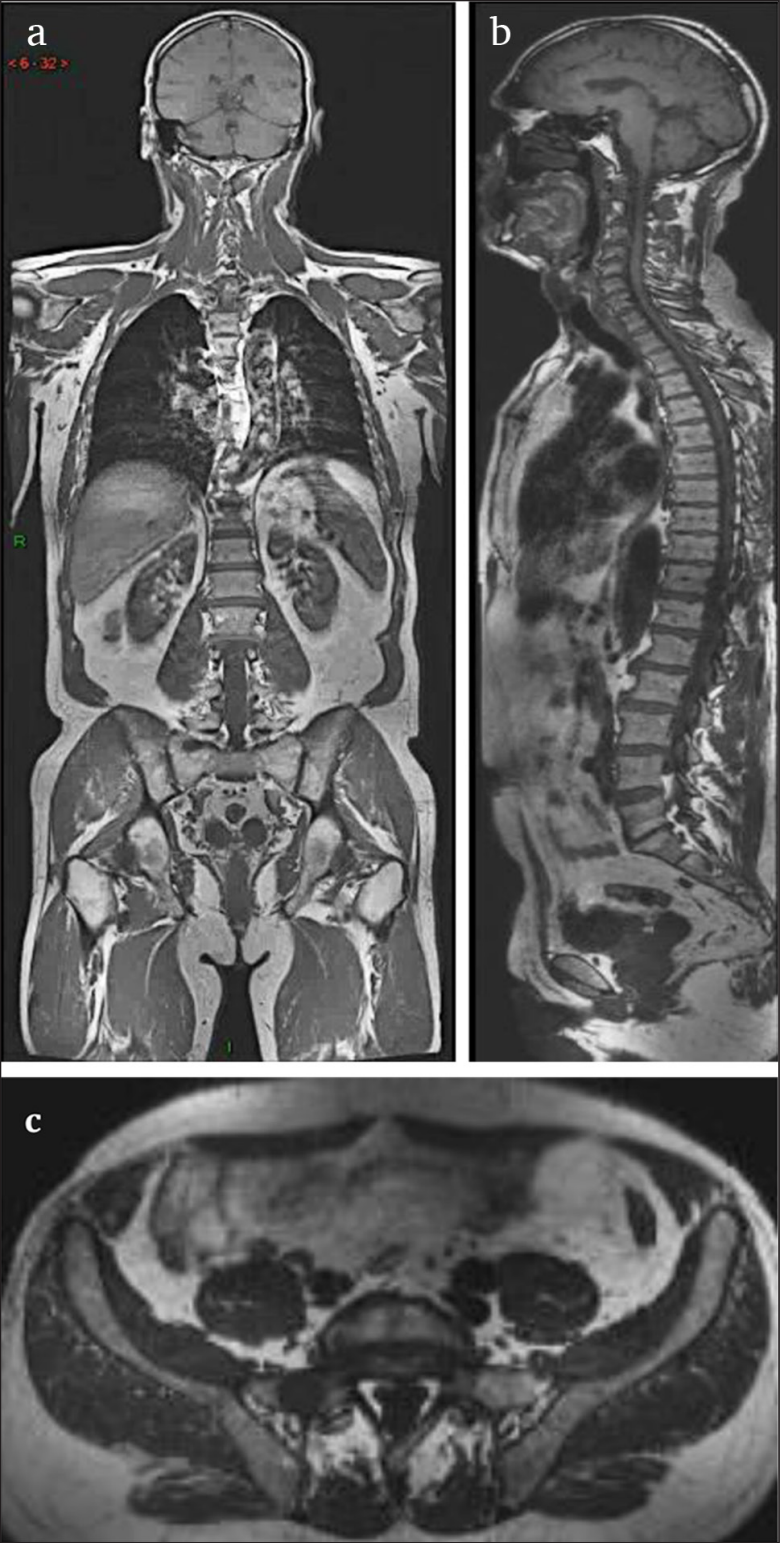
3D T1 anatomic MRI sequence reconstructed in coronal (a), sagittal (b) and axial plane (c).
